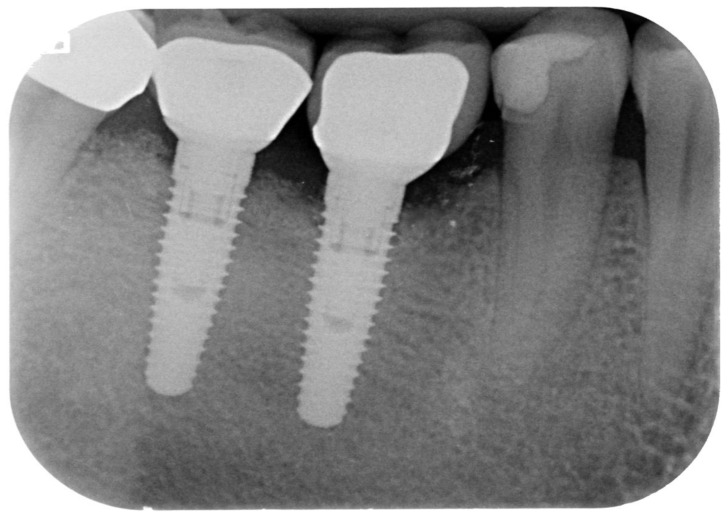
Follow-up radiograph (38 months).
